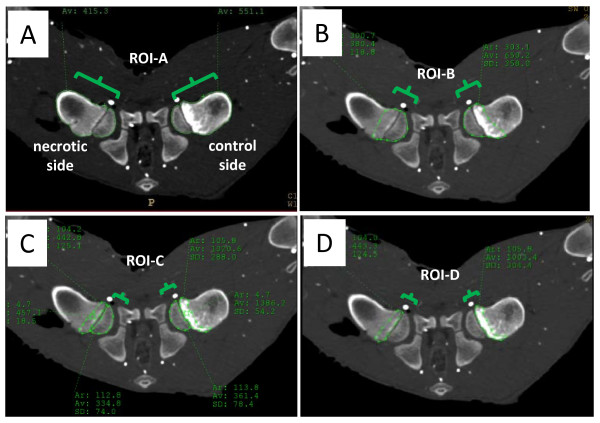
Cross-sectional CT image highlighting four regions of interest. (A) ROI-A: femoral head and neck. (B) ROI-B: whole femoral head. (C) ROI-C: proximal femoral head. (D) ROI-D: base of the femoral head.
